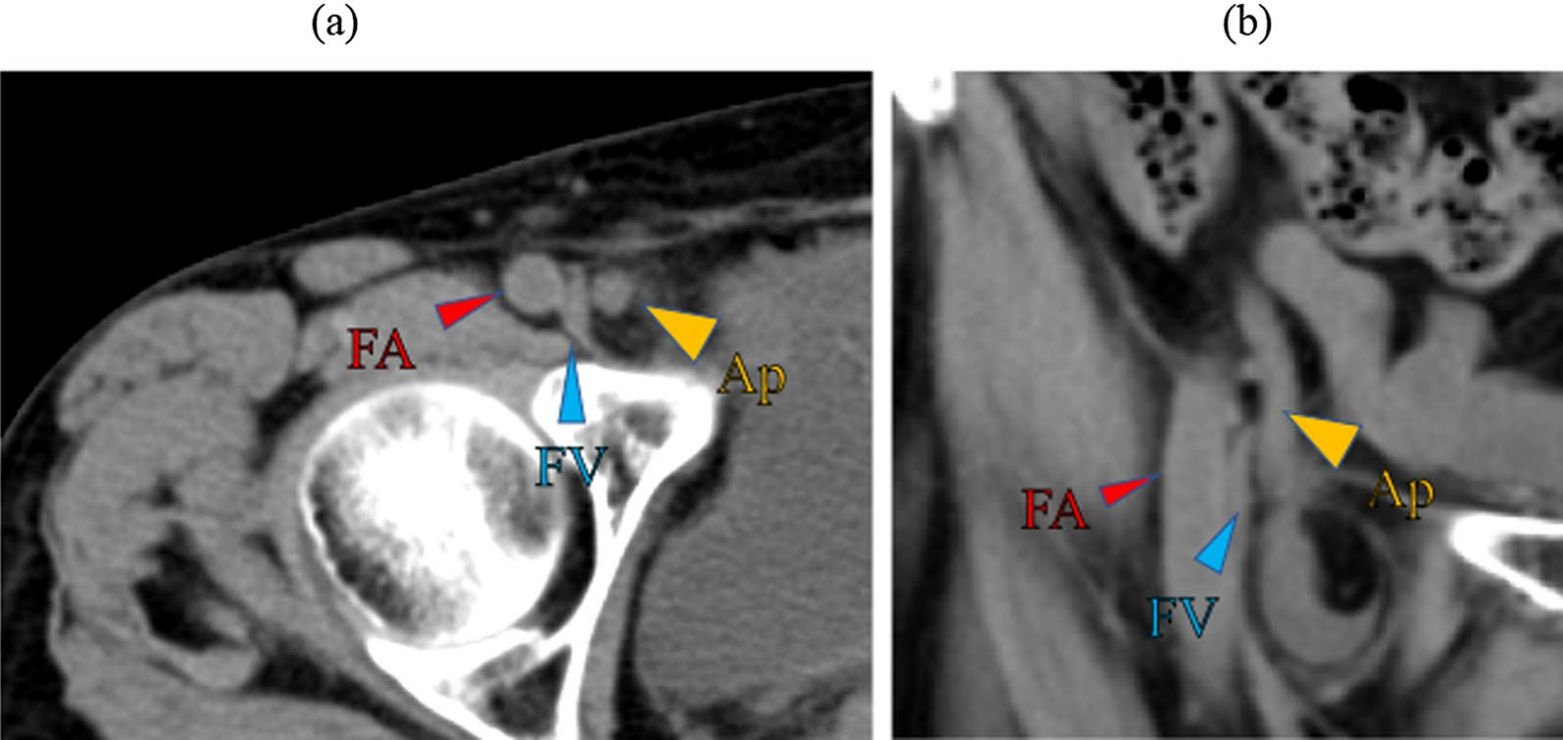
Computed tomography (Case 1). a Axial image showing a well-defined isodense structure (Ap) on the medial side of the right femoral vein (FV). b Coronal image showing an isodense blind-ended tubular structure (Ap) on the medial side of the right FV protruding from the abdominal cavity. *Ap* appendix, *FA* femoral artery
